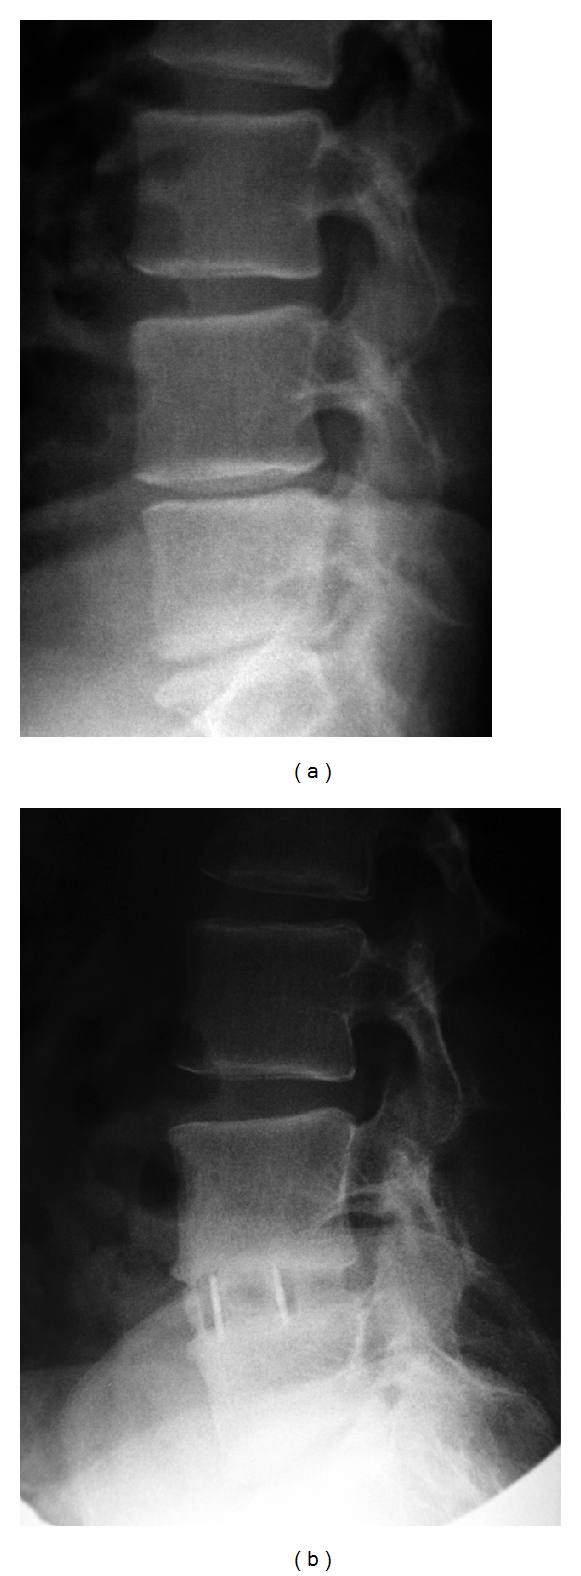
Case example number 2. Male, 58 years old, long history of lumbar axial pain and recurrent crisis event. VAS scores-preoperative 6; 1-week 3; 24-month 1. Patient underwent an L4L5 stand-alone lateral interbody fusion using rh-BMP. (a) Preoperative lateral orthostatic X-ray (b) 12-month lateral orthostatic X-ray.
